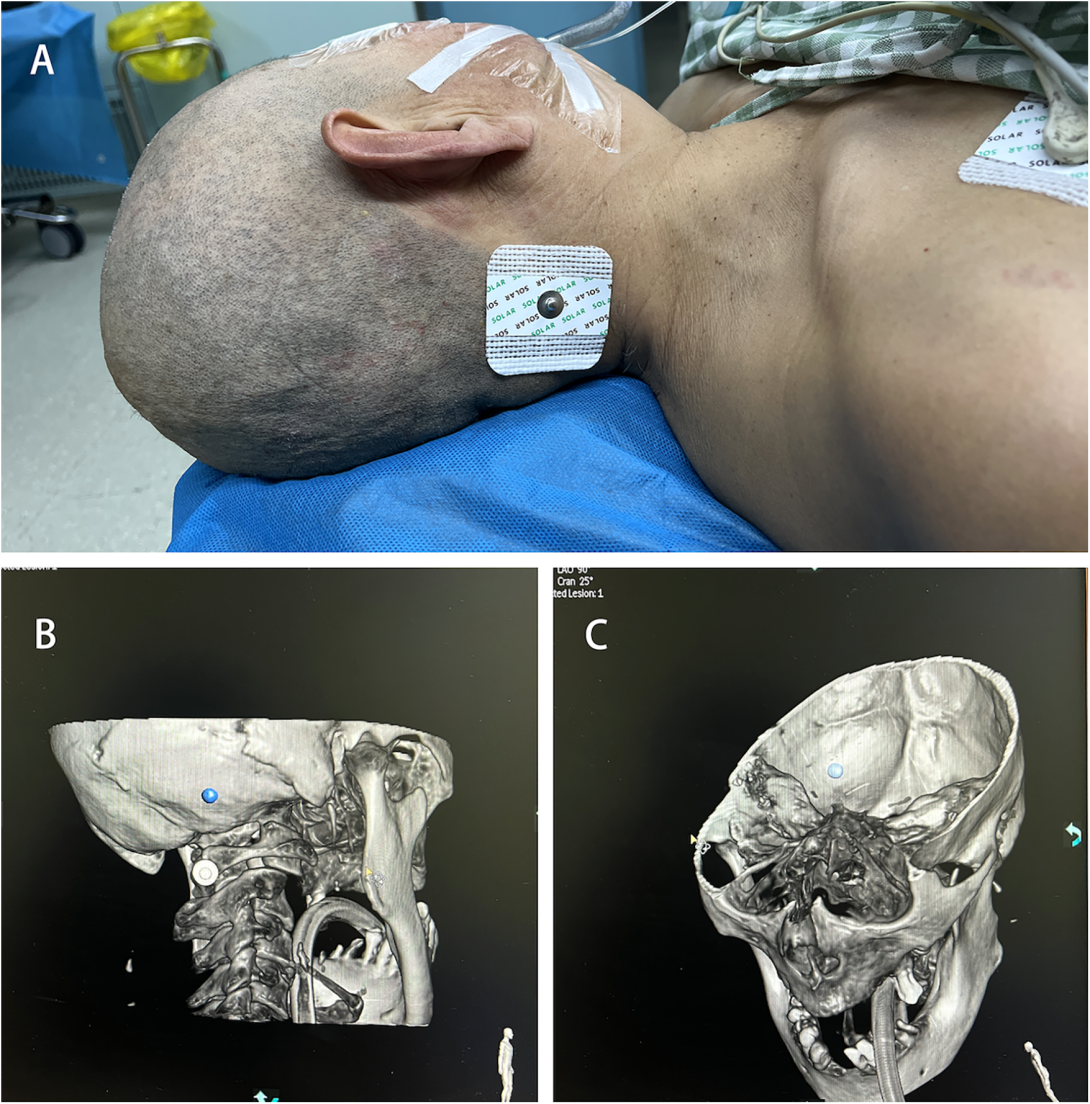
Puncture site marking. (A) Pre-puncture point. (B) C-arm CT scan marking bone hole position. (C) Avoidance of transverse sinus and sigmoid sinus groove.
